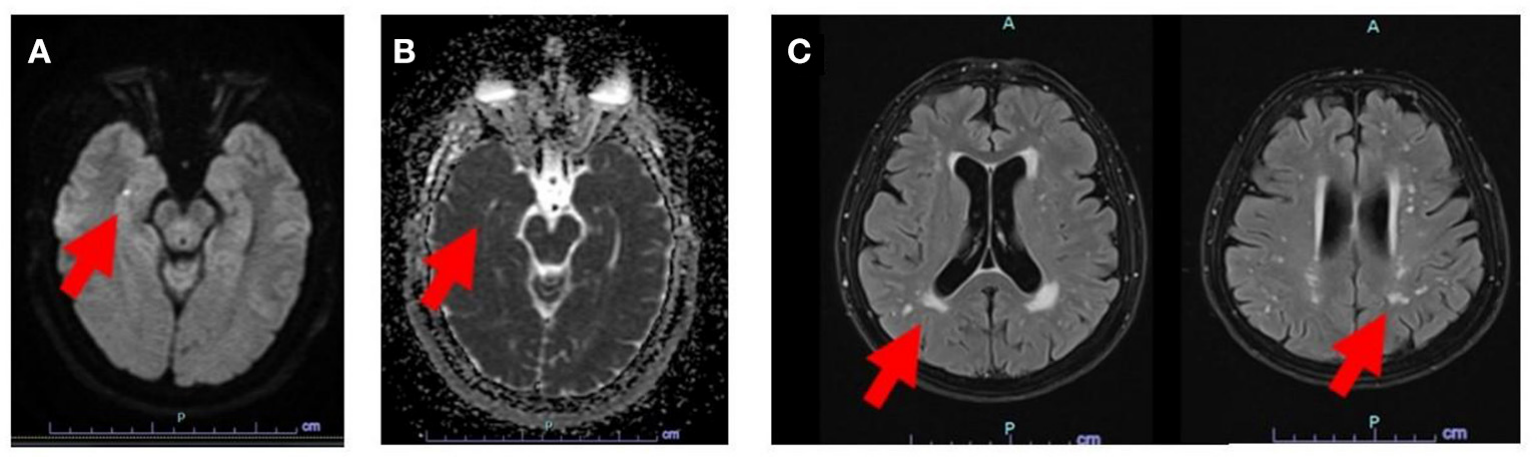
MRI image showing DWI hyperintense lesion in the right hippocampus (A), also seen by the corresponding ADC maps which display diffusion restriction (B). Subcortical FLAIR lesions were also found in the same patient with a Fazekas score 2 (C). Red arrows point to specific lesions.
